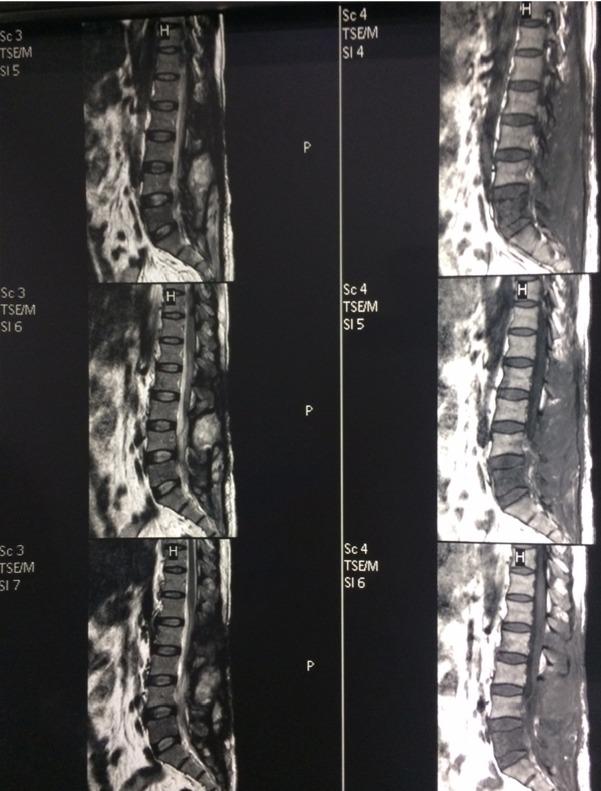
Two-month follow-up MRI showing complete abscess resolution and neural decompression but low signal of L5 in T1 (a) and high signal inT2 (b) indicating L5 spondylitis.
